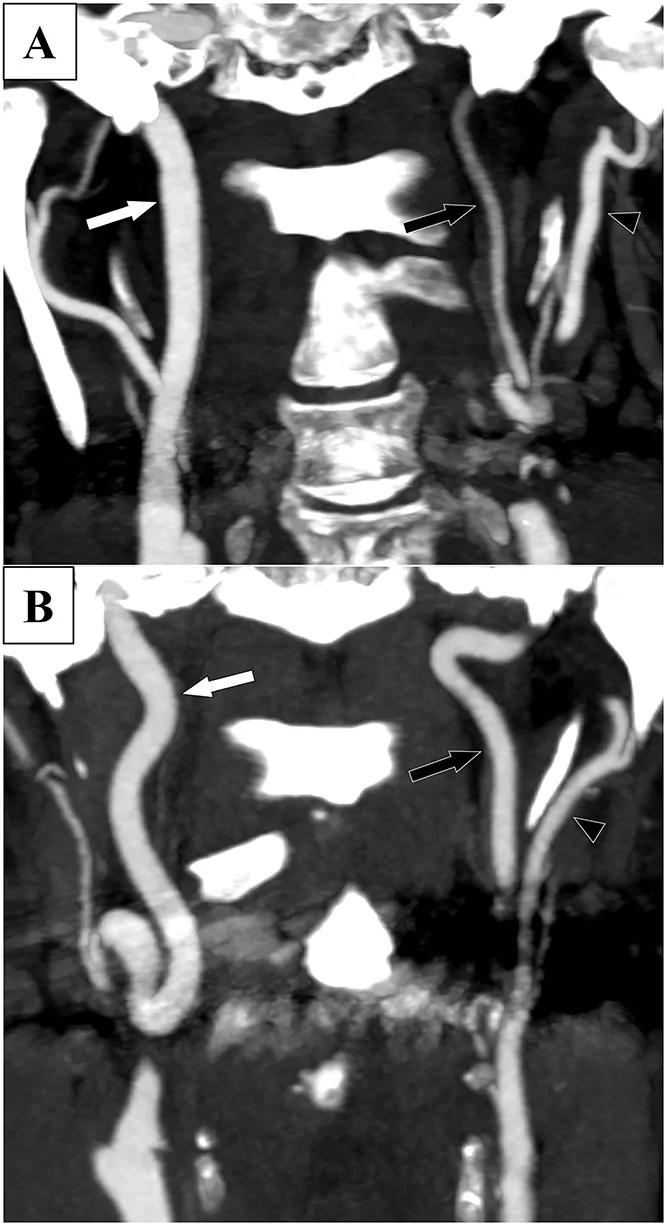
Two examples of left-sided carotid near-occlusion on CTA, coronal views. (A) With full collapse. Distal to a severe stenosis (not in plane), the distal ICA is very small (1.1 mm diameter, white arrow), clearly smaller than the contralateral ICA (5.4 mm, black arrow) and the ipsilateral ECA (2.4 mm, arrowhead). (B) Without full collapse. Distal to a severe stenosis (not in plane), the distal ICA is small (2.9 mm diameter, white arrow), clearly smaller than the contralateral ICA (4.6 mm, black arrow) and of similar diameter to the ipsilateral ECA (2.5 mm, arrowhead). Abbreviations: ECA = external carotid artery; ICA = internal carotid artery.
